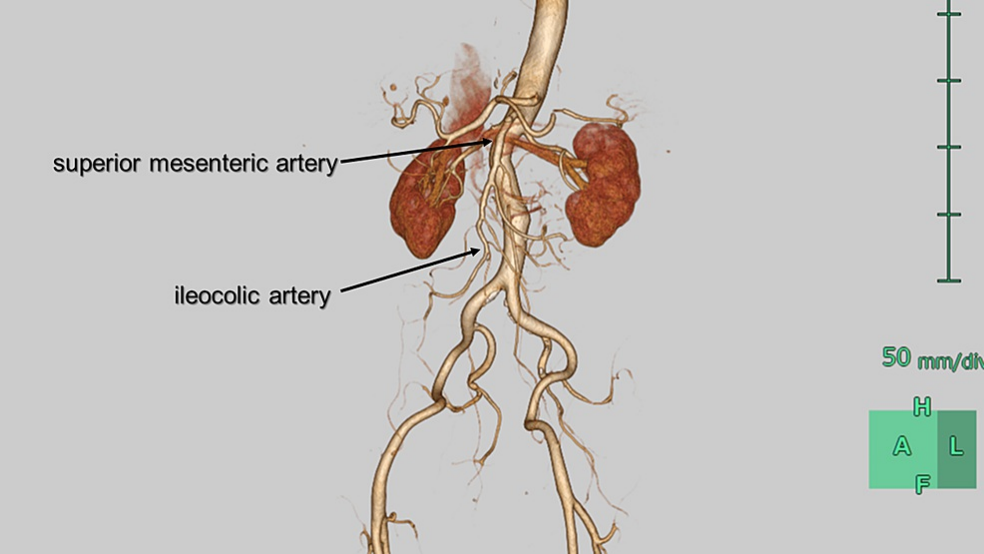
3D computed tomography of superior mesenteric artery four days after the second admission Better visualization of peripheral branches of superior mesenteric artery.
